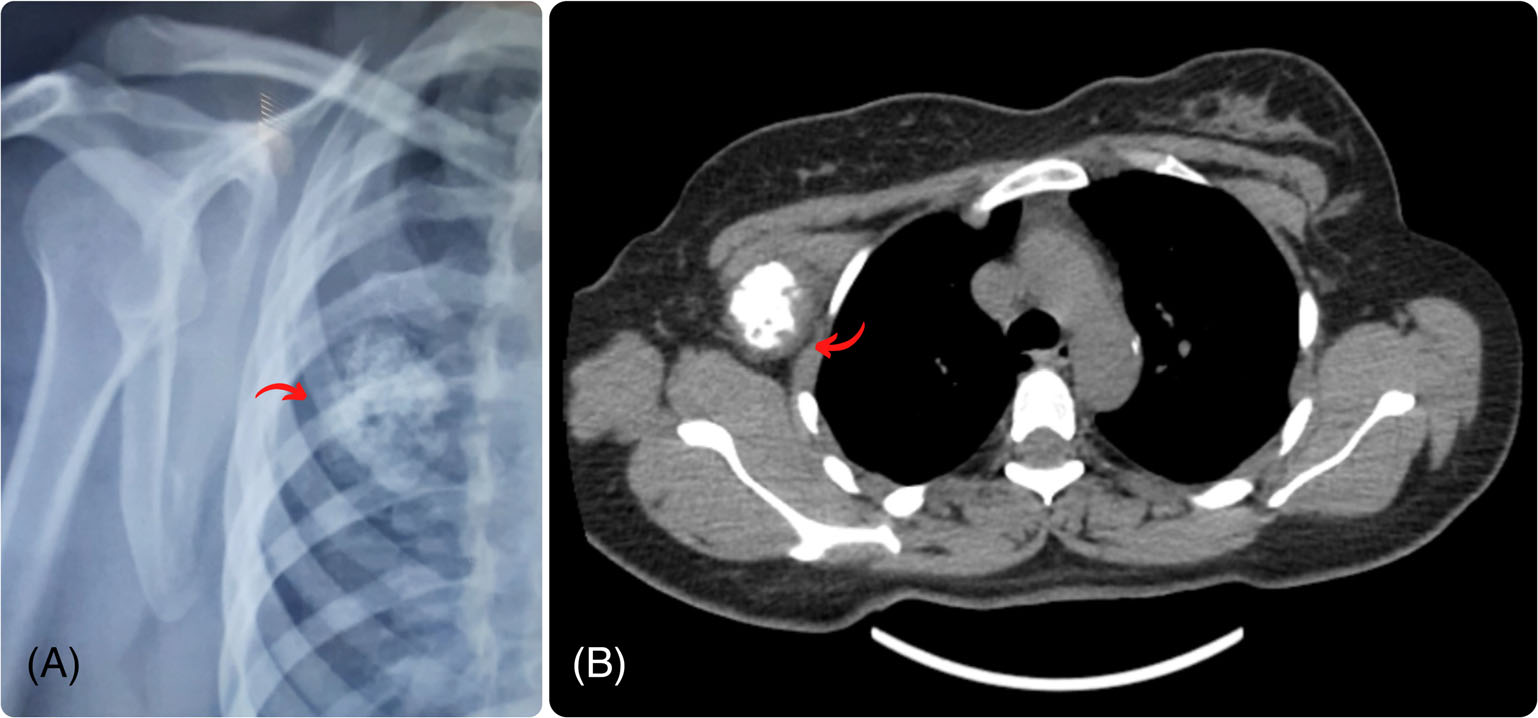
Primary soft tissue chest wall mesenchymal chondrosarcoma with biphasic pattern in a 38‐year‐old woman. (A) Axial non‐contrast CT shows a mass (arrow) in the right hemithorax under pectoralis muscle and inseparable from the second rib with a distinct calcified (central) and non‐calcified (periphery) components. (B) The chest X‐ray determined a well‐defined mass with intralesional rings and arcs calcification which is the near ribs.
